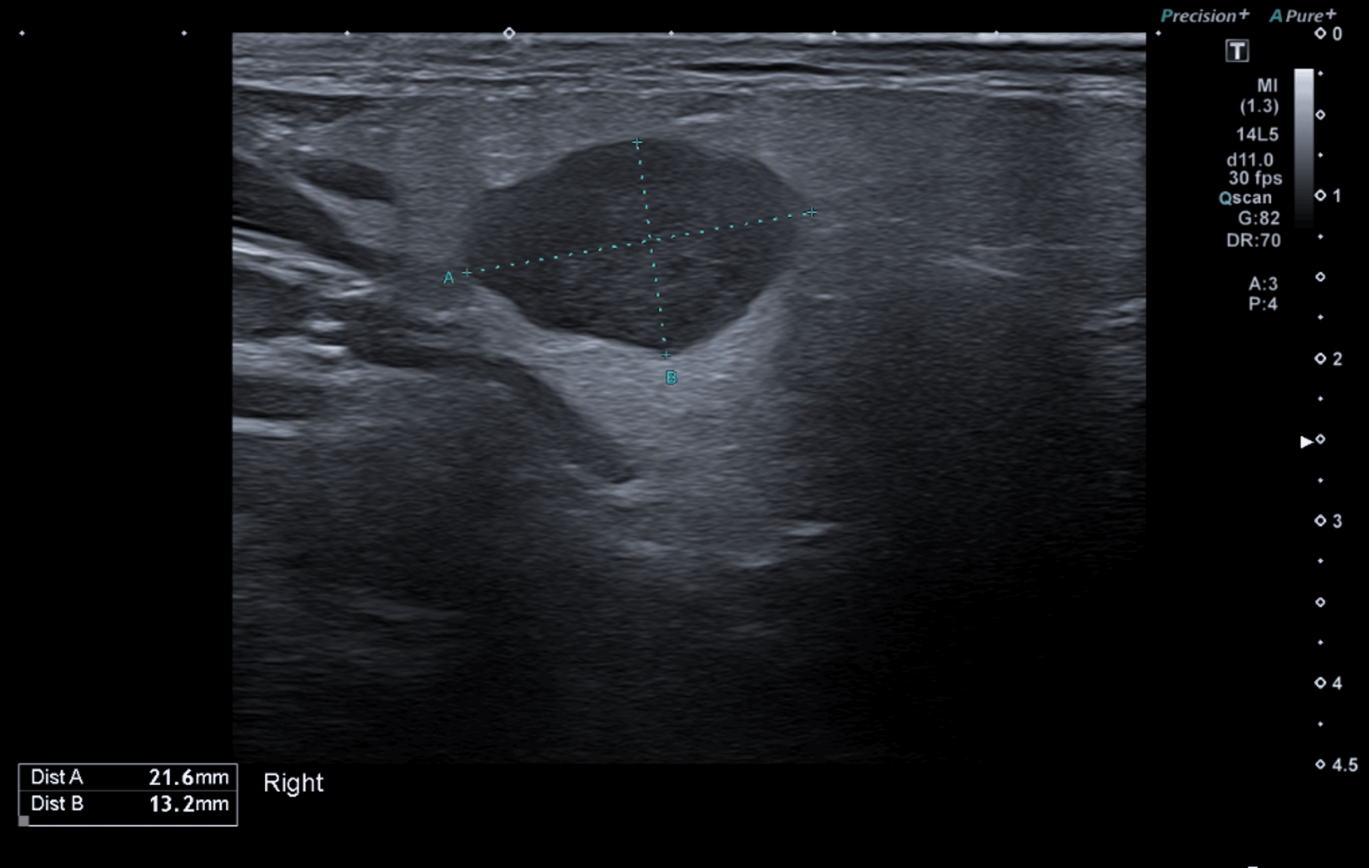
US showing parotid lesion US: ultrasound
